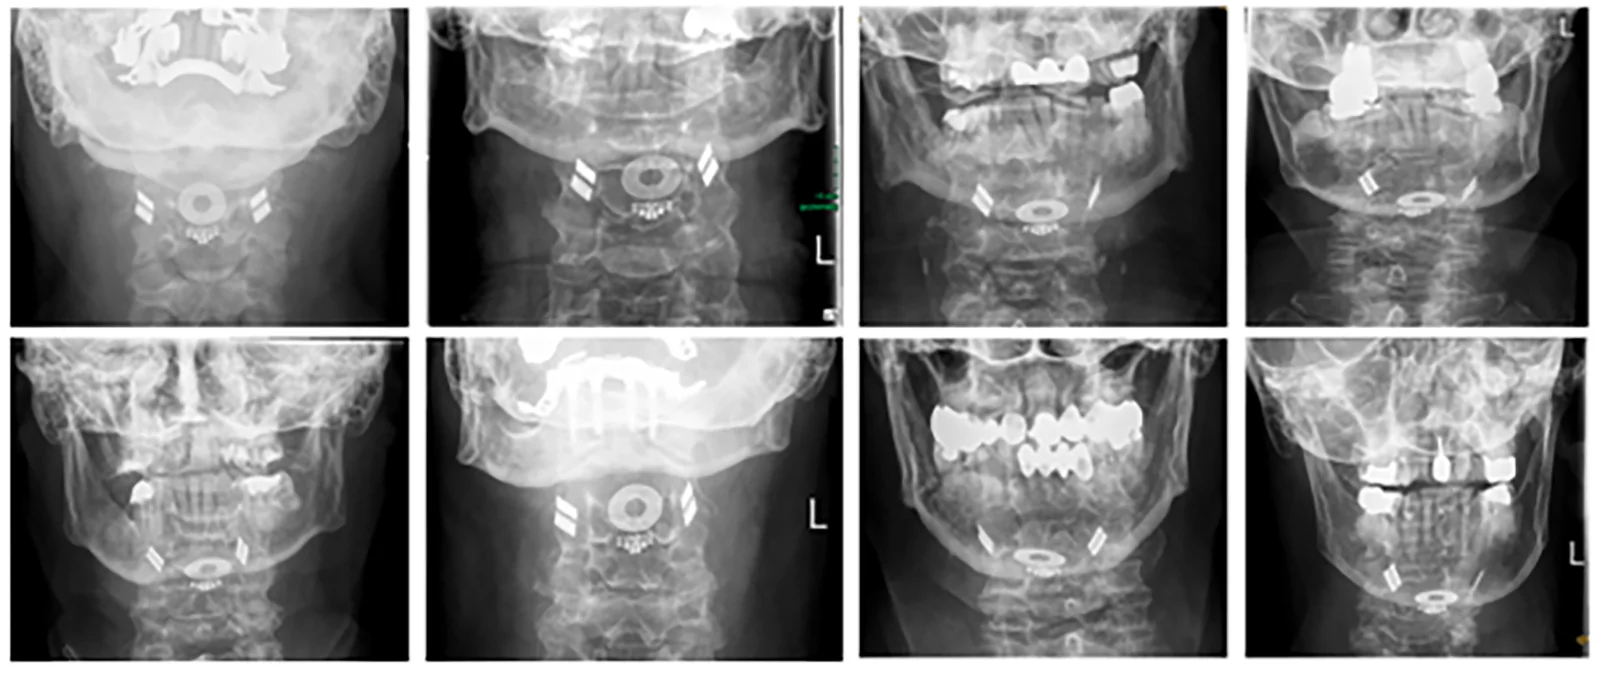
x-ray (a.p.). Symmetrical alignment of bilateral paddle electrodes in 8 patients.
